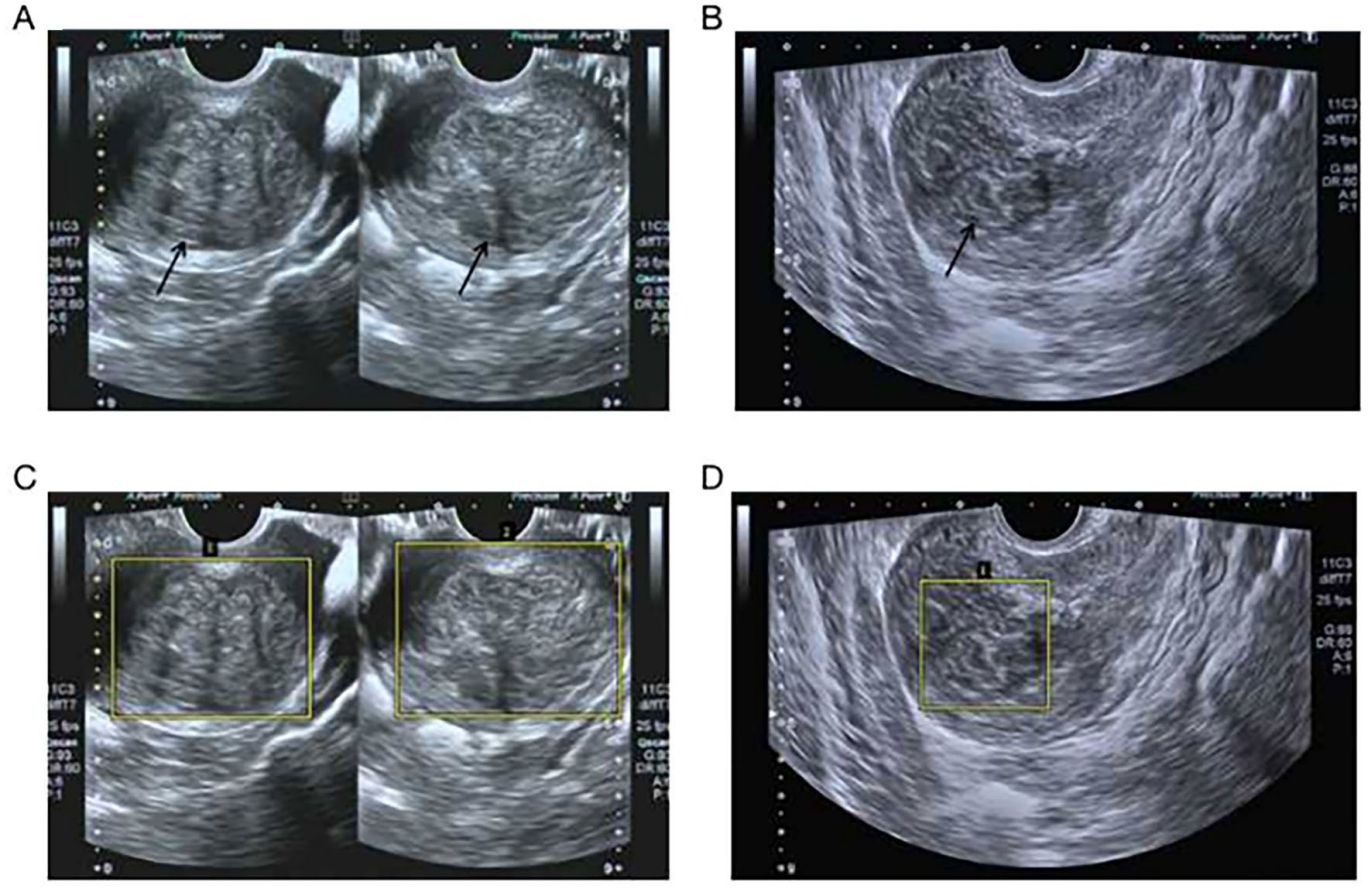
Examples of original ultrasound data and sample data after labeling. (A) Training sample of ultrasound image with two uterine fibroids. (B) Training sample of ultrasound image with one fibroid. (C) Labeled training sample of ultrasound image with two uterine fibroids. (D) Labeled training sample of an ultrasound image with only one fibroid. The yellow box shows the extent of fibroids marked by the ultrasonographers.
